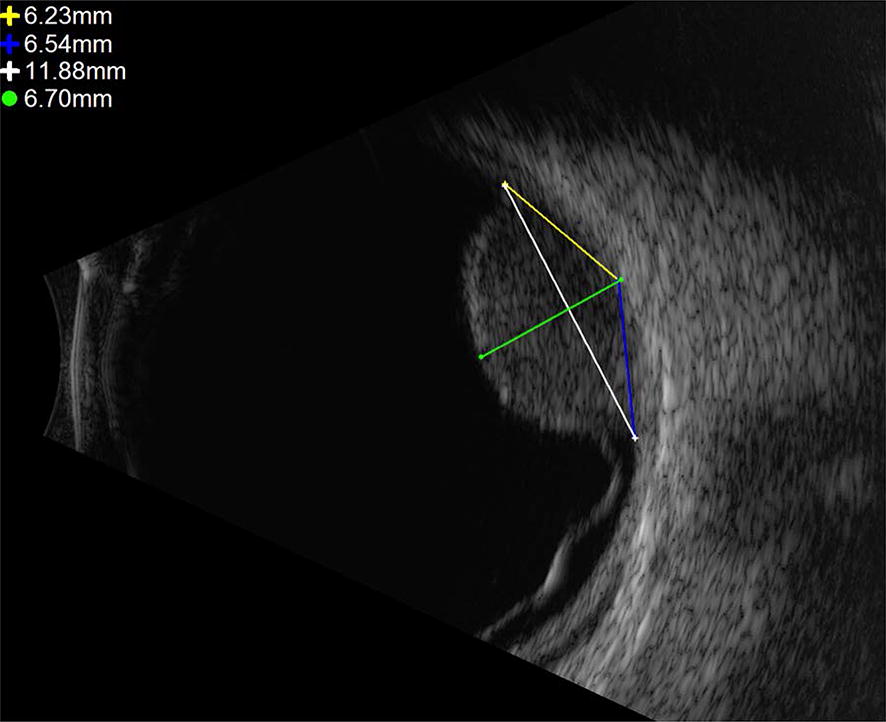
Relationship between one-chord method and two-chord method on B scan ultrasound
